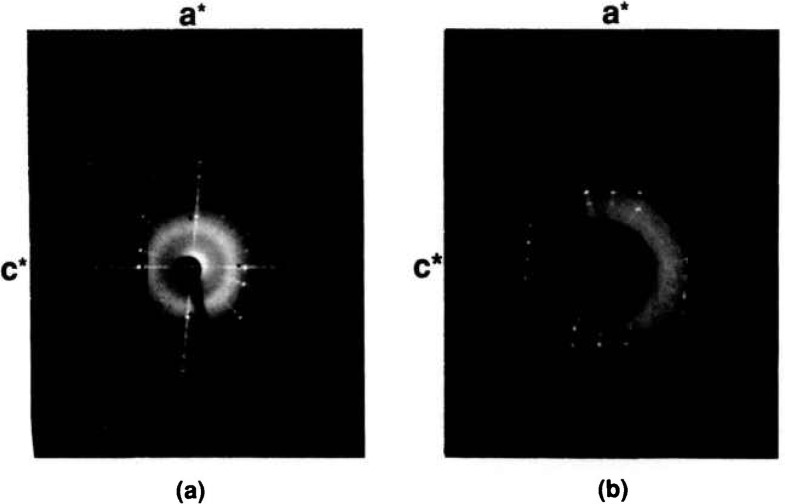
X-ray precession photographs for SrBi_2_O_4_ (a) *h0l*, (b) *hIl.*
